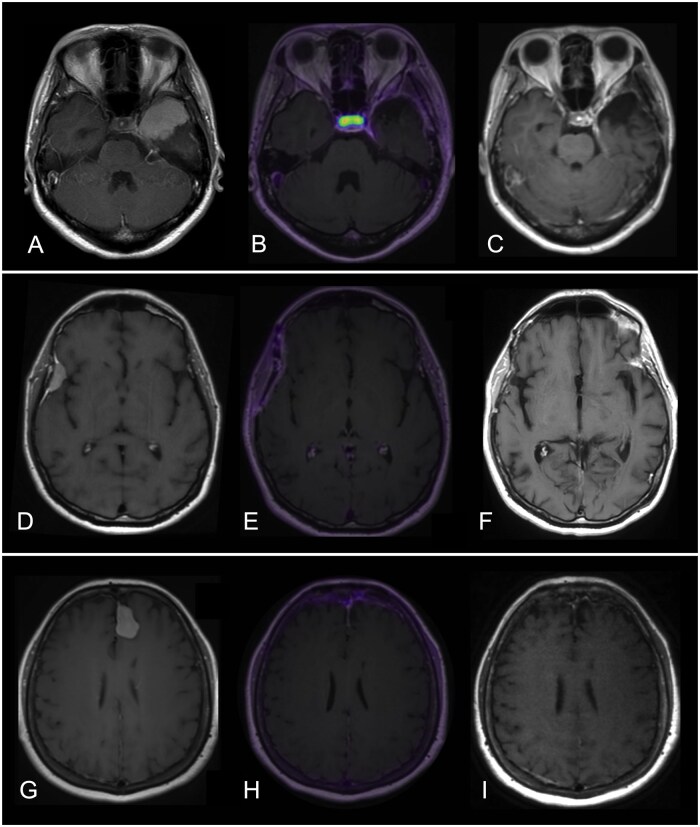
Three representative patients with WHO grade 2 meningiomas. A 36-year-old woman presented with headache, nausea, and blurry vision and was found to have an extra-axial dural-based homogeneously enhancing mass arising from the left greater sphenoid wing, measuring 4.2 × 4.0 cm with associated mass effect on the left medial frontal lobe (A). Pathology demonstrated CNS WHO grade 2 meningioma (6 mitoses per 10 high-power-fields, Ki-67: 8%). Post- operative follow-up MRI and DOTATATE PET/MRI (B) performed 3 months after resection demonstrated no evidence of residual or recurrent tumor. Smooth linear dural thickening and enhancement was visualized along the left anterior temporal convexity and left superior tentorial leaflet, with mild DOTATATE avidity below diagnostic threshold for meningioma (SUV 2.5, SUVRsss 1.7), favored to be reactive. Moderate physiologic avidity in the pituitary gland is consistent with expected SSTR2 expression. Serial MRI demonstrated interval decrease in linear dural thickening/enhancement, compatible with expected evolution of post-operative changes. Most recent follow-up MRI performed 3.8 years postoperatively (C) showed no evidence of residual or recurrent tumor. A 55-year-old man who presented with memory impairment was found to have an extra-axial dural-based homogeneously enhancing right frontotemporal convexity mass (D) which had demonstrated growth on serial MRI examinations, measuring 2.1 × 1.0 cm in the axial plane on preoperative MRI. Pathology demonstrated CNS WHO grade 2 meningioma (10 mitotic figures per 10 high-power-fields, Ki-67: 10%). Post- operative follow-up MRI and DOTATATE PET/MRI (E) performed 4 months after resection demonstrated no evidence of residual or recurrent meningioma; there was smooth linear dural thickening and enhancement subjacent to the craniotomy site, with mild DOTATATE avidity below diagnostic threshold for meningioma (SUV 3.0, SUVRsss 2.5), favored to be reactive. The most recent follow-up MRI performed 5.3 years postoperatively (F) demonstrates no evidence of residual or recurrent tumor. A 58-year-old woman who presented with memory loss was found to have an extra-axial dural-based homogeneously enhancing mass arising from the left aspect of the anterior falx cerebri, measuring 2.7 × 1.8 cm with associated mass effect on the left medial frontal lobe (G). Pathology demonstrated CNS WHO grade 2 meningioma (4 mitotic figures per 10 high-power-fields, Ki-67: 7%). Post- operative follow-up MRI and DOTATATE PET/MRI (H) performed 10 months after resection demonstrated no evidence of residual or recurrent meningioma; there was smooth linear dural thickening and enhancement along the left frontal convexity and anterior falx, surrounding the anterior third of the SSS without narrowing or occlusion. Corresponding DOTATATE avidity was mild and below diagnostic threshold for meningioma (SUV 2.7, SUVRsss 2.7), favored to be reactive. The most recent follow-up MRI performed 5.0 years postoperatively (I) showed no evidence of residual or recurrent tumor. PET/MRI fused images are displayed using the PET Rainbow color scale, windowed from 0 to 15 maximum SUV.
